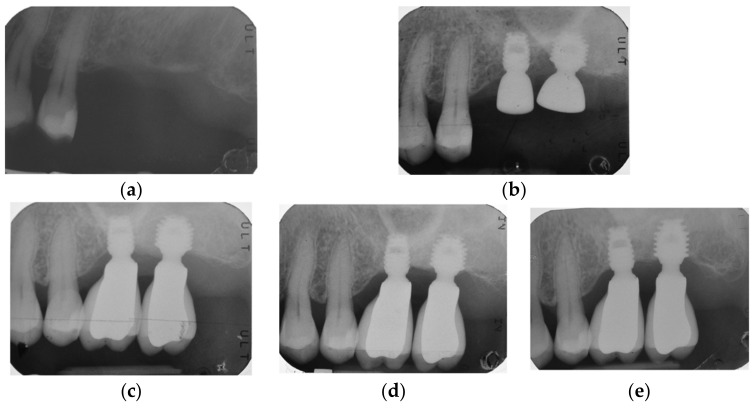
Single implants placed in 2.6 and 2.7 sites (4.5 × 6 mm and 5 × 6 mm): (a) pre-operative radiograph before implant placement; see minimal bone levels (2.6 RCBH = 4.5 mm; 2.7 RCBH = 4.7 mm); (b) radiograph obtained at time of placement; see augmented sinus floor (2.6 sinus lift = 2.3 mm; 2.7 sinus lift = 2 mm); (c) radiograph obtained at time of loading; (d) radiograph obtained at 3-year follow-up; (e) radiograph obtained at 5-year follow-up. See stable bone levels with minimal changes between 3-year (2.6 RCBH = 5.2 mm; 2.6 sinus lift = 1 mm; 2.7 RCBH = 5.55 mm; 2.7 sinus lift = 1.3 mm) and 5-year follow-up (2.6 RCBH = 5 mm; 2.6 sinus lift = 1 mm; 2.7 RCBH = 5.35 mm; 2.7 sinus lift = 1.15 mm).
